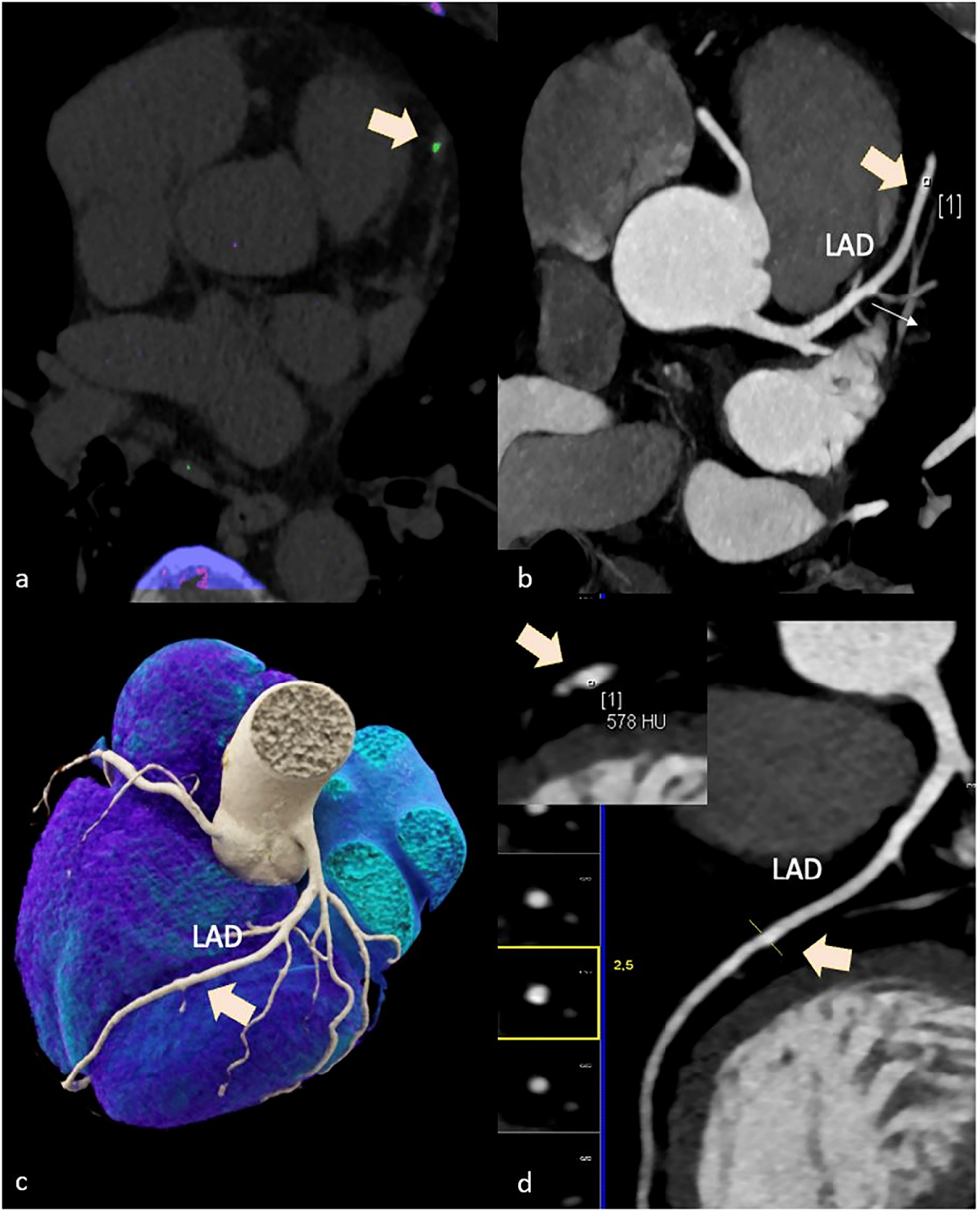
A 74-year-old male with gout (a) and a small coronary plaque with an equivalent CT density (578 HU) to the coronary lumen (arrow in b) in the distal LAD artery, which was difficult to visualize and correlated with an MSU-positive lesion on DECT (arrow in a). Green = MSU-positive lesion on DECT scan (a); coronary CTA (b) Three-dimensional volume rendering technique (c) and curved multiplanar reconstruction (d). In this patient, the CAC score was minimal with 3.4 Agatston units. CAC, Coronary calcium; CTA, Computed tomography angiography; DECT, Dual-energy computed tomography; LAD, Left ascending coronary artery; MSU, Monosodium urate
